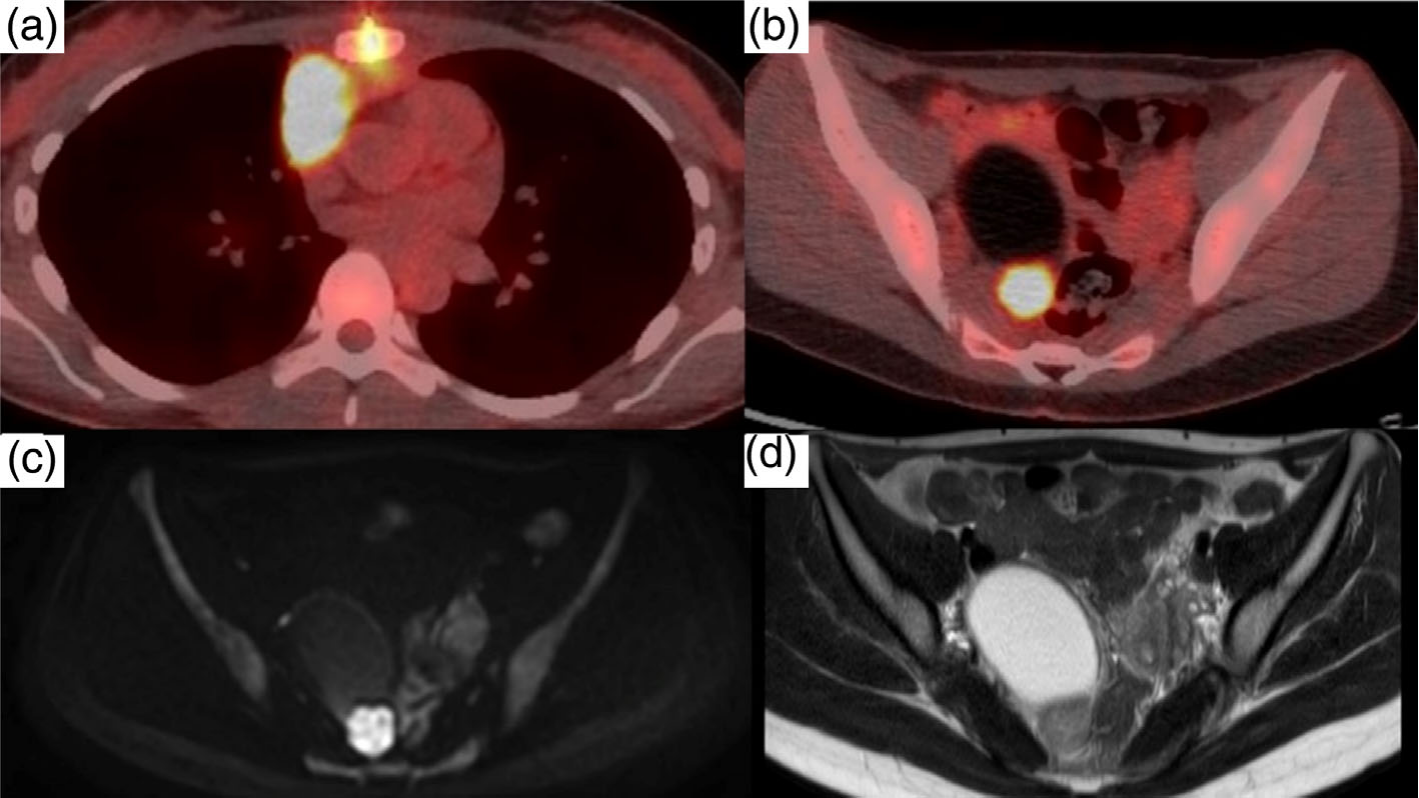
(a) Fluorodeoxyglucose‐positron emission tomography of the thorax in a horizontal section. (b) Fluorodeoxyglucose‐positron emission tomography of the pelvic region in a horizontal section. (c) Diffusion‐weighted magnetic resonance imaging of the right ovarian cyst in a horizontal section. (d) T2‐weighted magnetic resonance imaging of the right ovarian cyst in a horizontal section.
